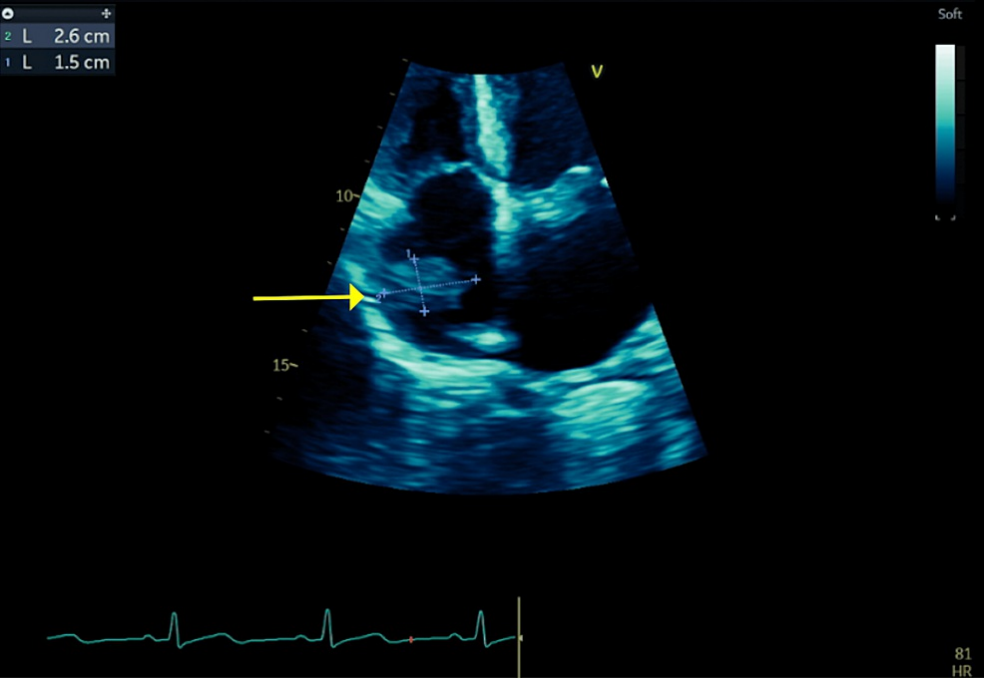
Transthoracic echocardiogram (TTE) image The yellow arrow shows a 2.6 cm by 1.5 cm right atrial mass without clear valvular vegetations.
